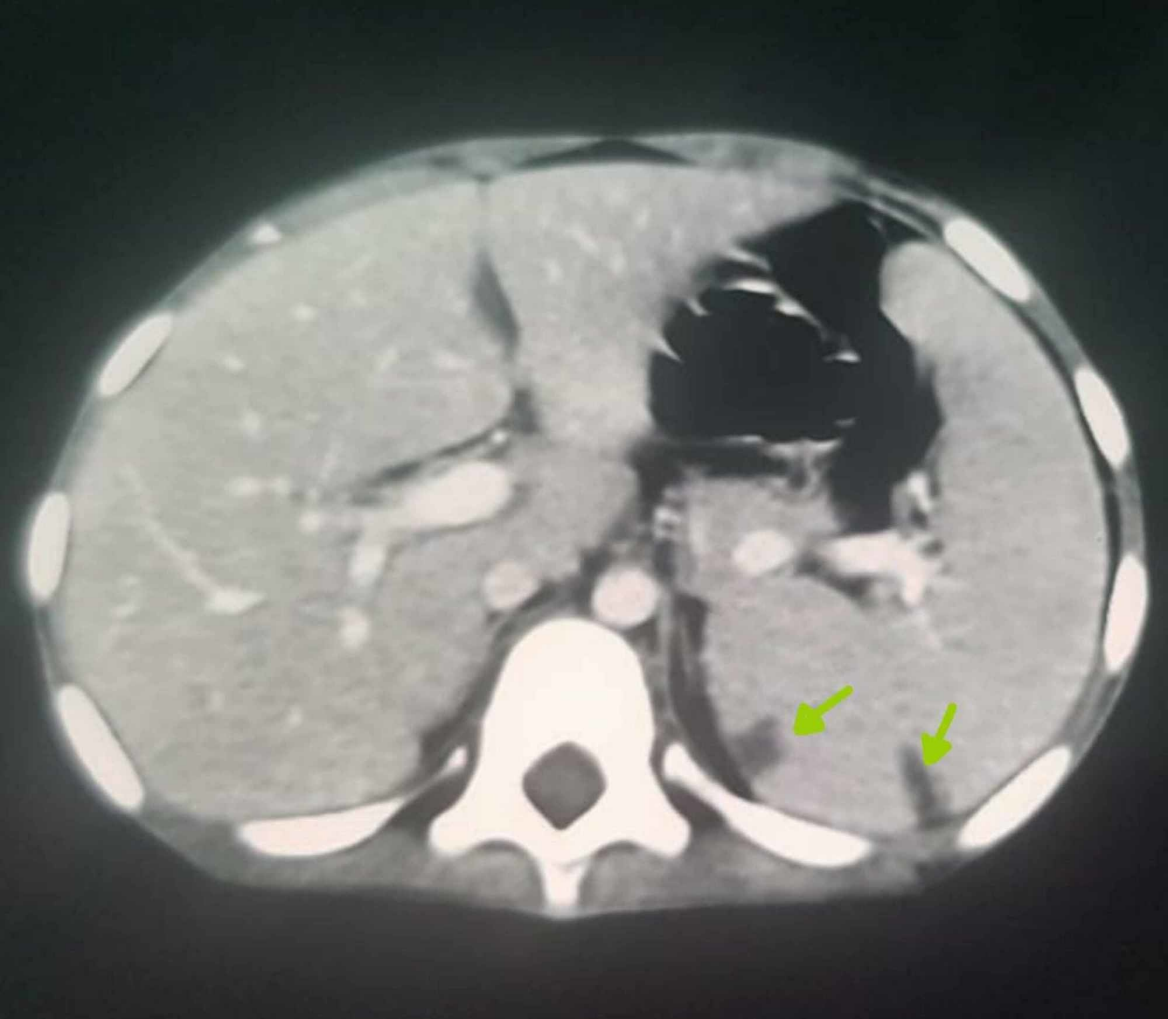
Axial computed tomography (CT) of abdomen with contrast. Axial CT of abdomen with contrast showing two subcapsular hypodense areas (shown by the arrows) signifying abscesses.
